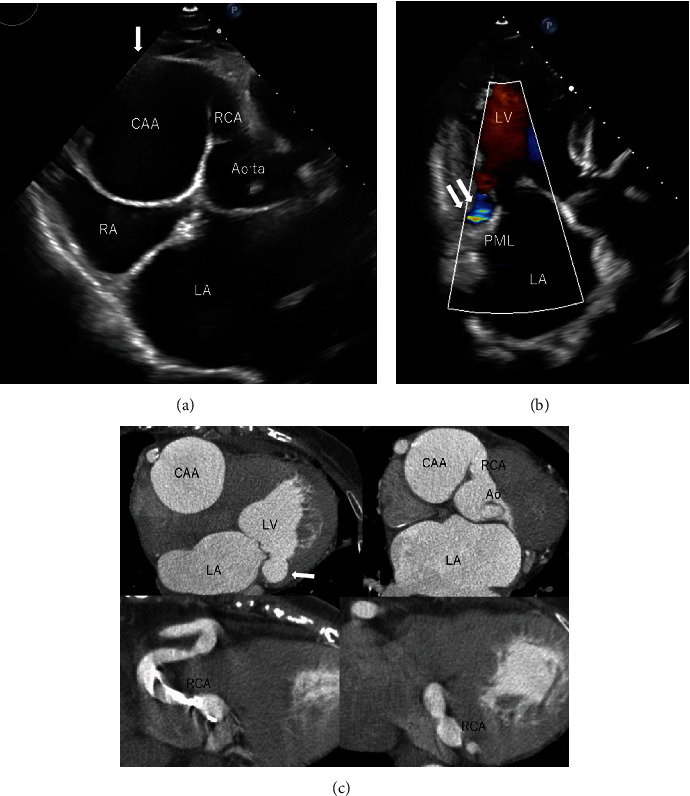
(a, b) Preoperative ultrasound echocardiography. CAA (↓) CAF (↓↓). (c) MDCT of coronary artery. CAF (↓). CAA: coronary artery aneurysm; RCA: right coronary artery; RA: right atrium; LA: left atrium; LV: left ventricle; PML: posterior mitral reflet; Ao: ascending aorta.
